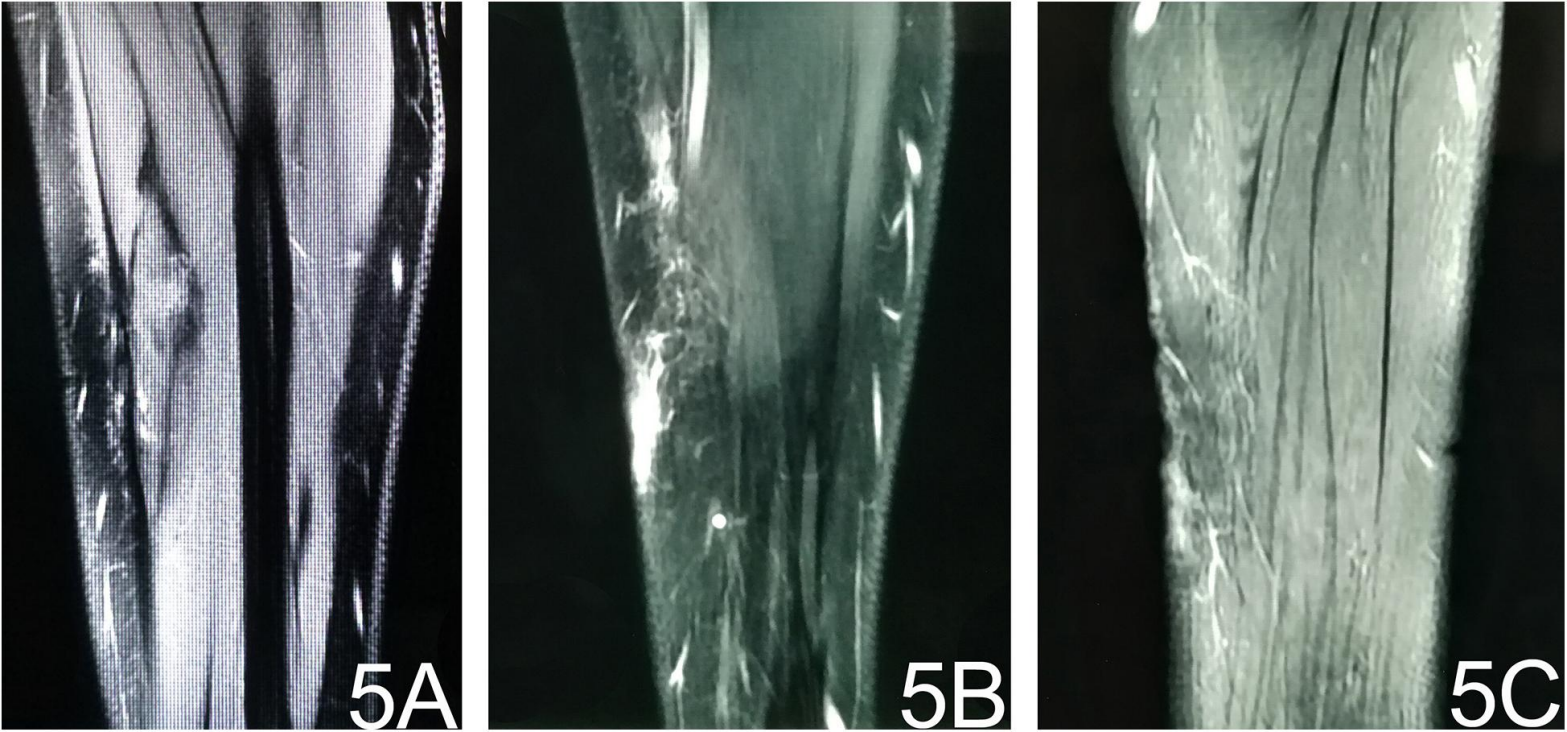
Post-operative MRI. (A) T1-weighted image reveals subcutaneous stripe- and patch-like hypointense areas at the operative site. (B) Fat-suppressed T2-weighted image shows corresponding hyperintense signal, indicating post-surgical oedema. (C) Contrast-enhanced fat-suppressed T1-weighted image demonstrates thin, patchy enhancement consistent with post-operative granulation tissue.
